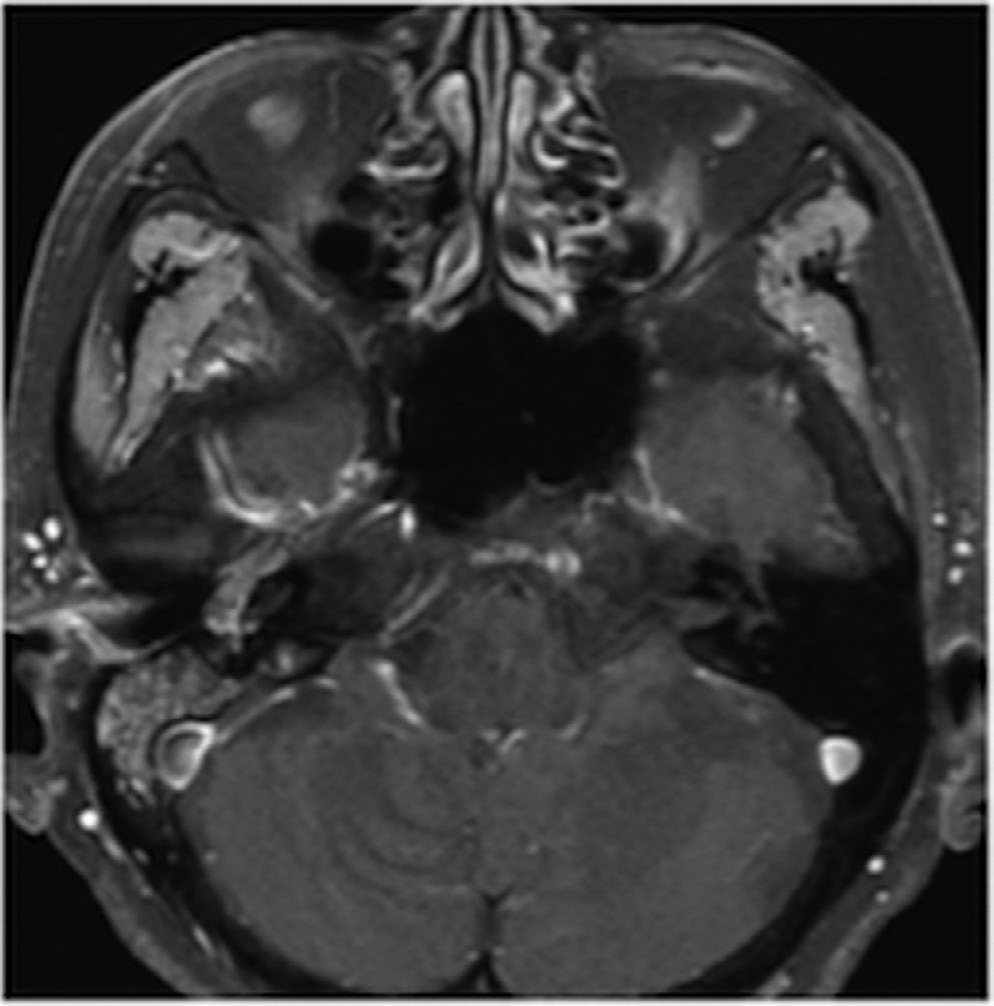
T1‐weighted axial MRI after eight courses of nivolumab. The tumor was limited to the middle ear cavity
